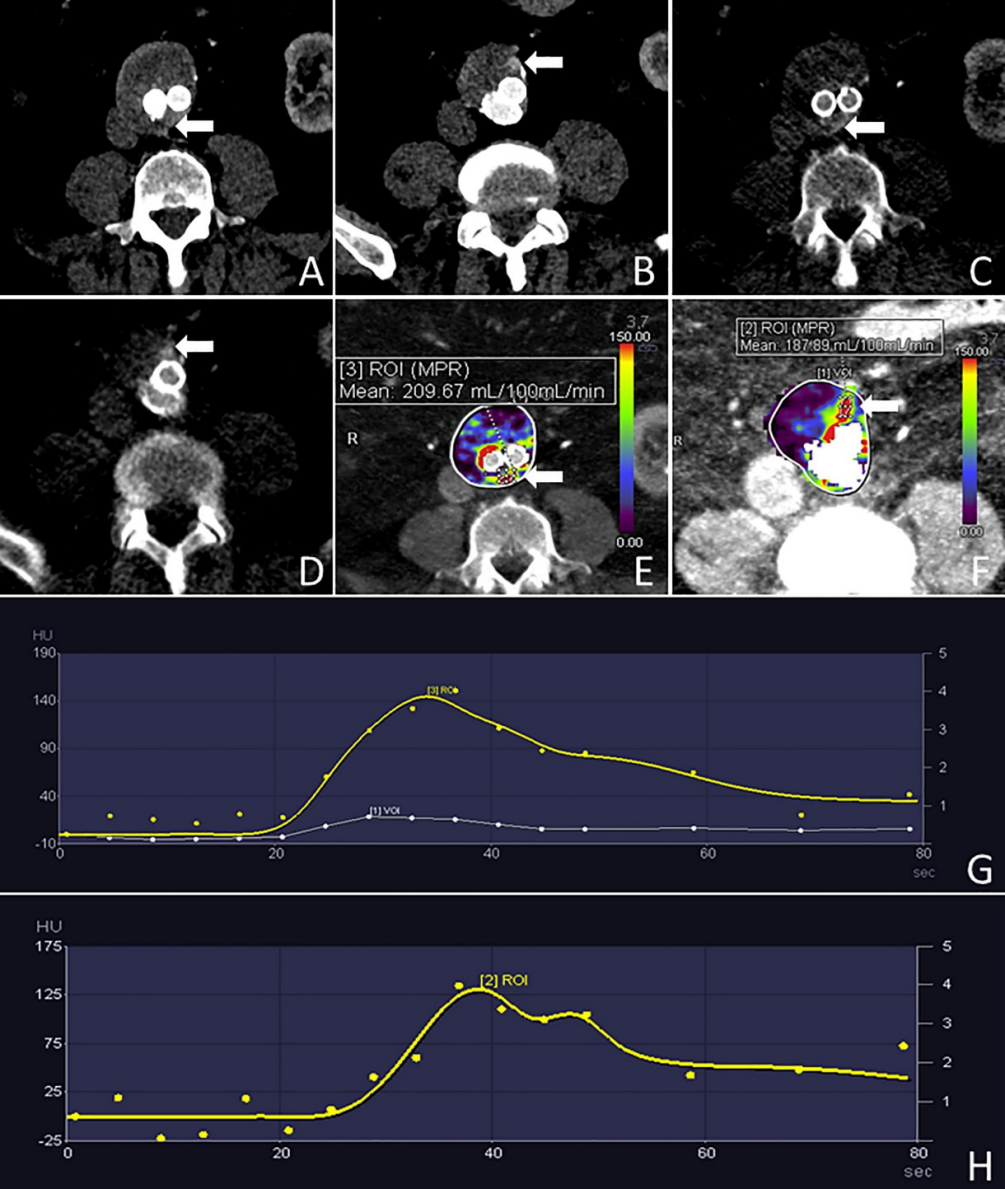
Case demonstration. 74-year-old man with a BMI of 33.51 kg/m^2^ who had undergone EVAR 1.5 years prior to the CTA examination. The arterial phase of the static CTA shows a type II endoleak fed by a lumbar artery and the inferior mesenteric artery (arrows in A and B, respectively). This is also depicted by the dCTA study during the acquisition performed at 38 seconds after contrast media injection (arrows in C and D). Panels E and F show the color-coded perfusion maps and the TACs (G and H). The TAC (G) corresponding to the cranial portion of the endoleak fed by a lumbar artery (arrow in E) shows a kinetic characterized by a rapid wash-in, a time to peak at 36 seconds, and a delayed washout. The TAC (H) corresponding to the caudal portion of the endoleak fed by the inferior mesenteric artery (arrow in F) shows a kinetic characterized by a rapid wash-in, two time to peaks at 38 and 48 seconds, and a delayed washout.
